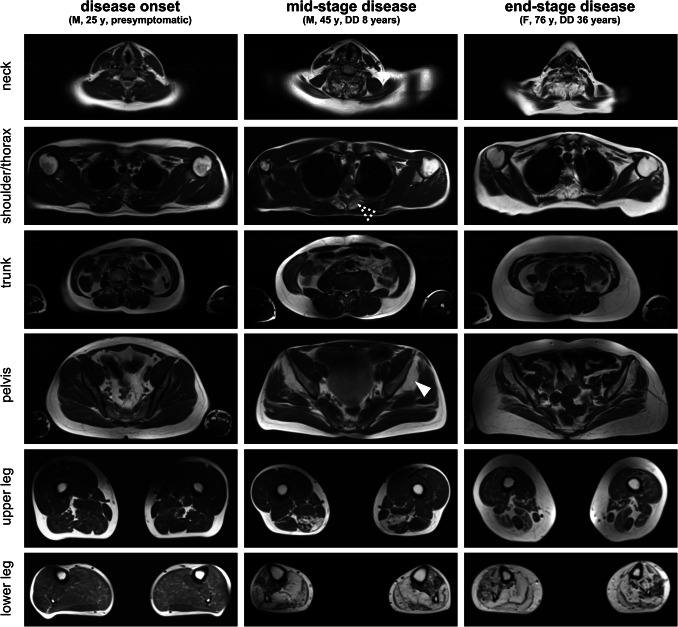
Magnetic resonance imaging in MATR3-associated distal myopathy. Representative T1-weighted MRI slices of head/neck, shoulder/thorax, trunk, pelvis, upper and lower leg in patients with different disease stages of MATR3-associated distal myopathy. Note the early affection of gluteus minimus muscle (white arrowhead) and thoracic segments of paraspinal musculature (dashed arrowhead) while gluteus medius and maximus muscles as well as cervical and lumbar segments of paraspinal musculature are relatively spared. *DD* disease duration, *y* years, *F* female, *M* male
